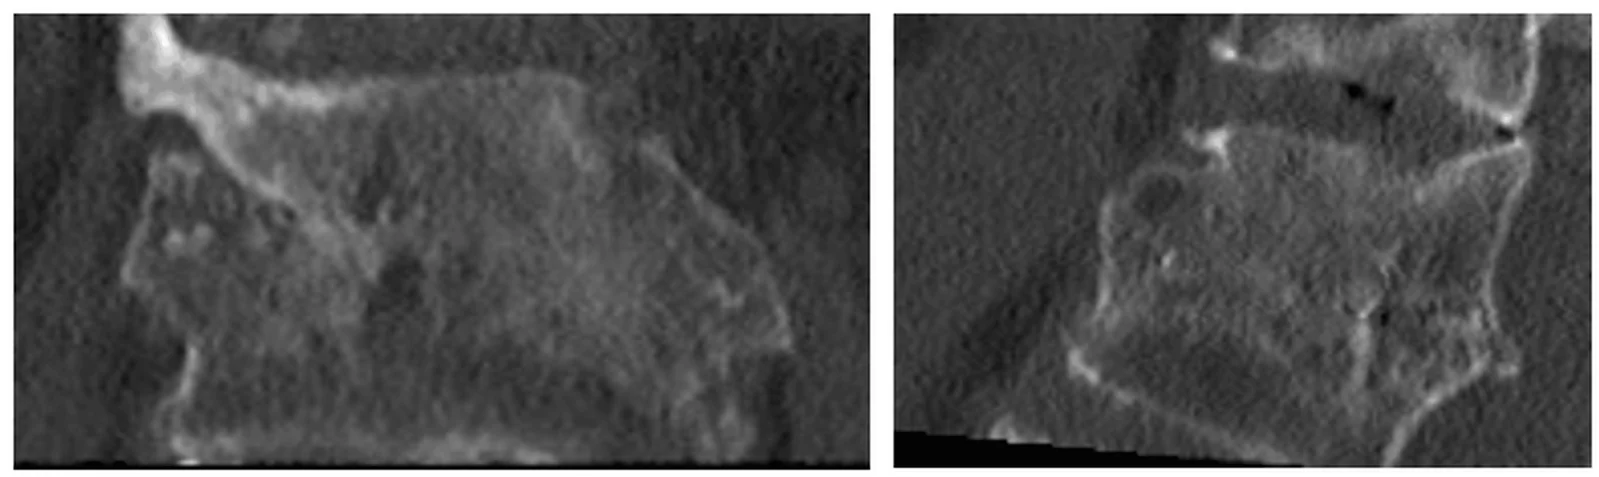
Postoperative CT at 12 months demonstrating bridging trabecular bone across the L1-L2 defect with continuity to the host endplates and no lucency, showing progression from the early consolidation seen at 8 months (Figure 5).
